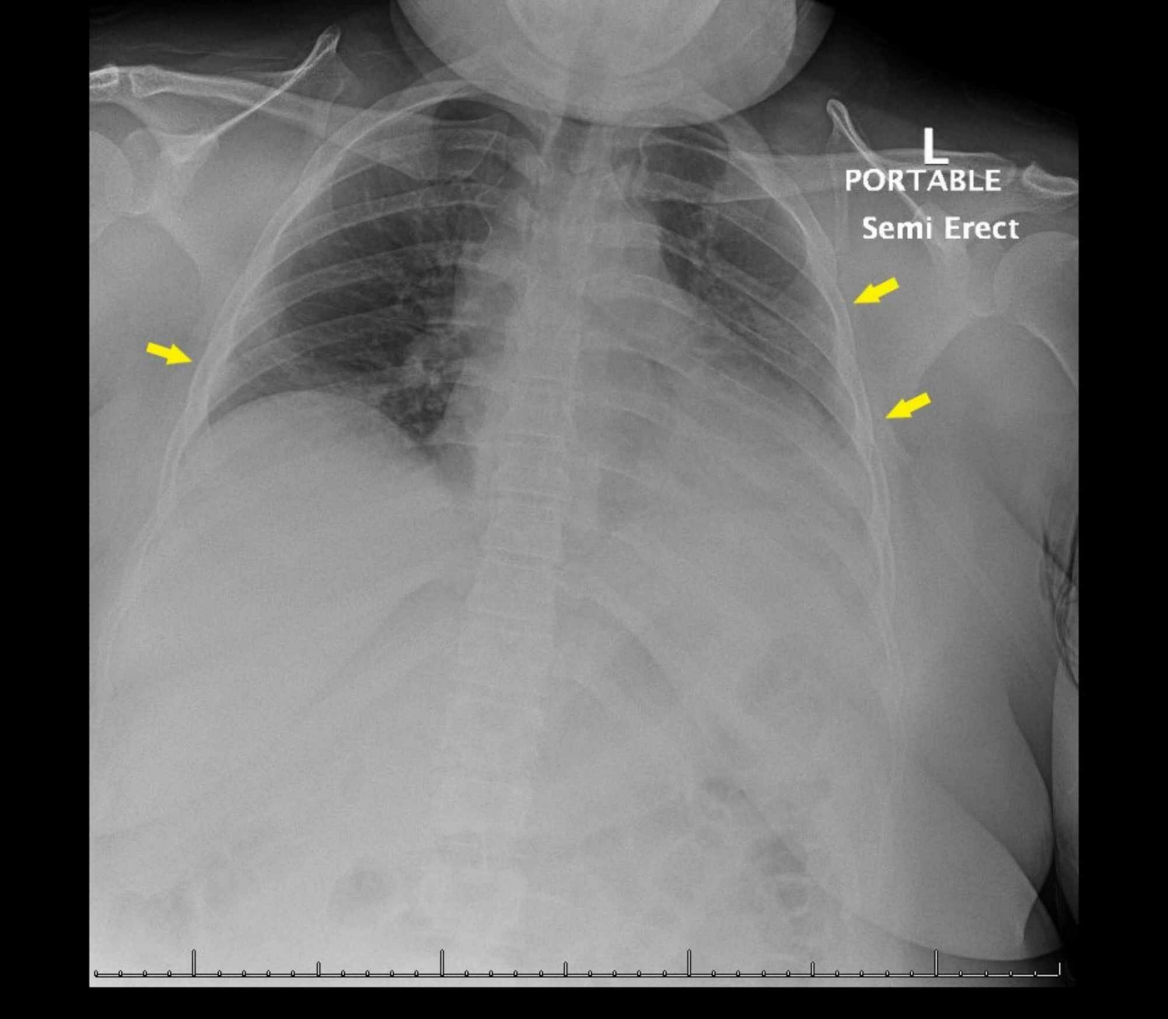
Initial chest radiograph with patchy opacities in the left mid to lower lung and right lung base suspicious for a multi-focal infectious process (arrows)
